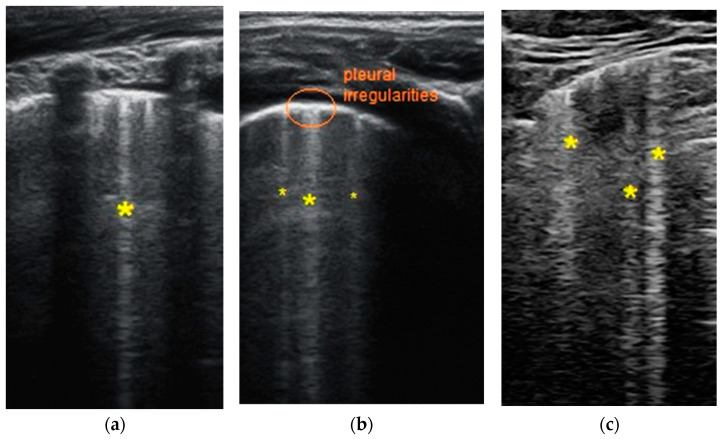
The lung ultrasound showed (a,c) sparse B-lines (yellow asterisk *) with small zones of pleural irregularities corresponding to a LUSS = 1 and (b) sparse B-lines (yellow asterisk) with small zones of pleural irregularities corresponding to a LUSS = 1.
